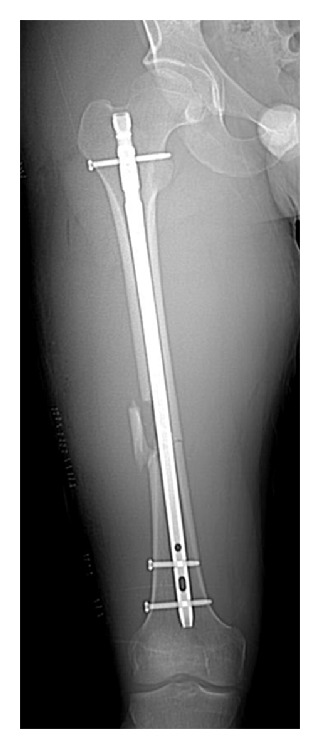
Anteroposterior CT scout image of the right femur. The cortical widths of the medial proximal and distal fragments appear to be equal.
